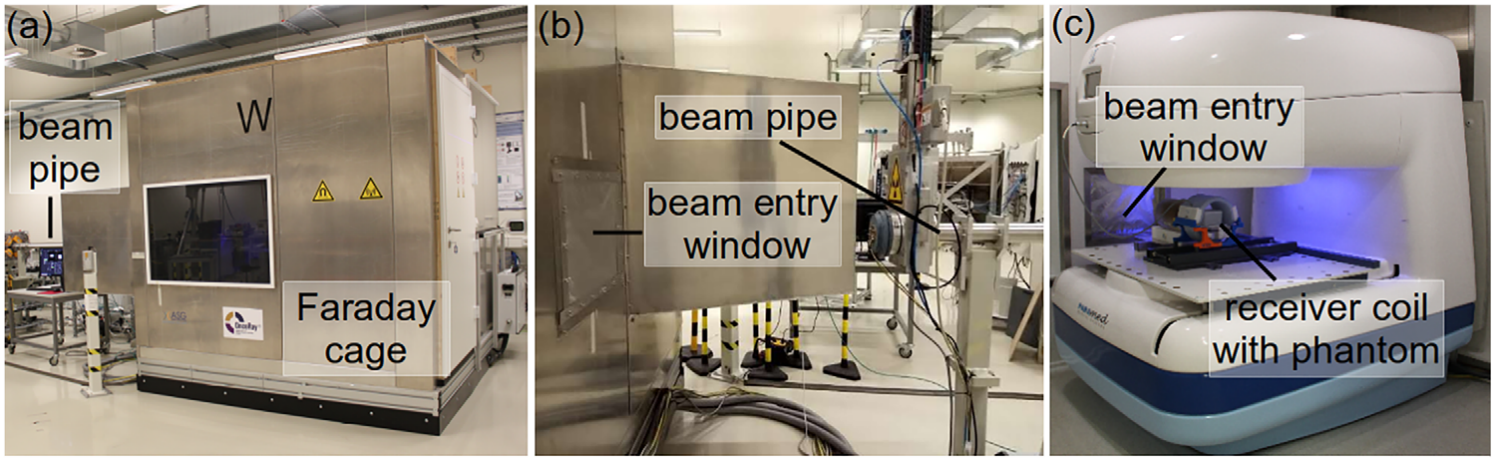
In‐beam MRI scanner positioned downstream of the horizontal proton research beamline. (a) The RF cabin hosts the scanner. (b) Closeup of the distal end of the beam pipe and the beam entry window of the RF cabin, taken from the opposite side. (c) The MRI scanner is located distally from the beam entry window inside the RF cabin. MRI, magnetic resonance imaging; RF, radiofrequency.
